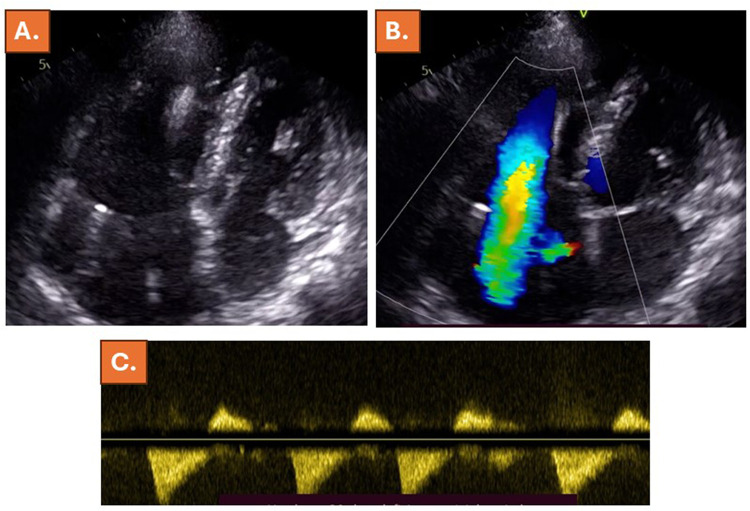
Echocardiography 2 weeks after the index presentation showed further worsening of the RV-function, accompanied by increased dilatation of the RV and D-shape of the LV (A), persistent torrential TR (B) with a triangular TR shape (C).
